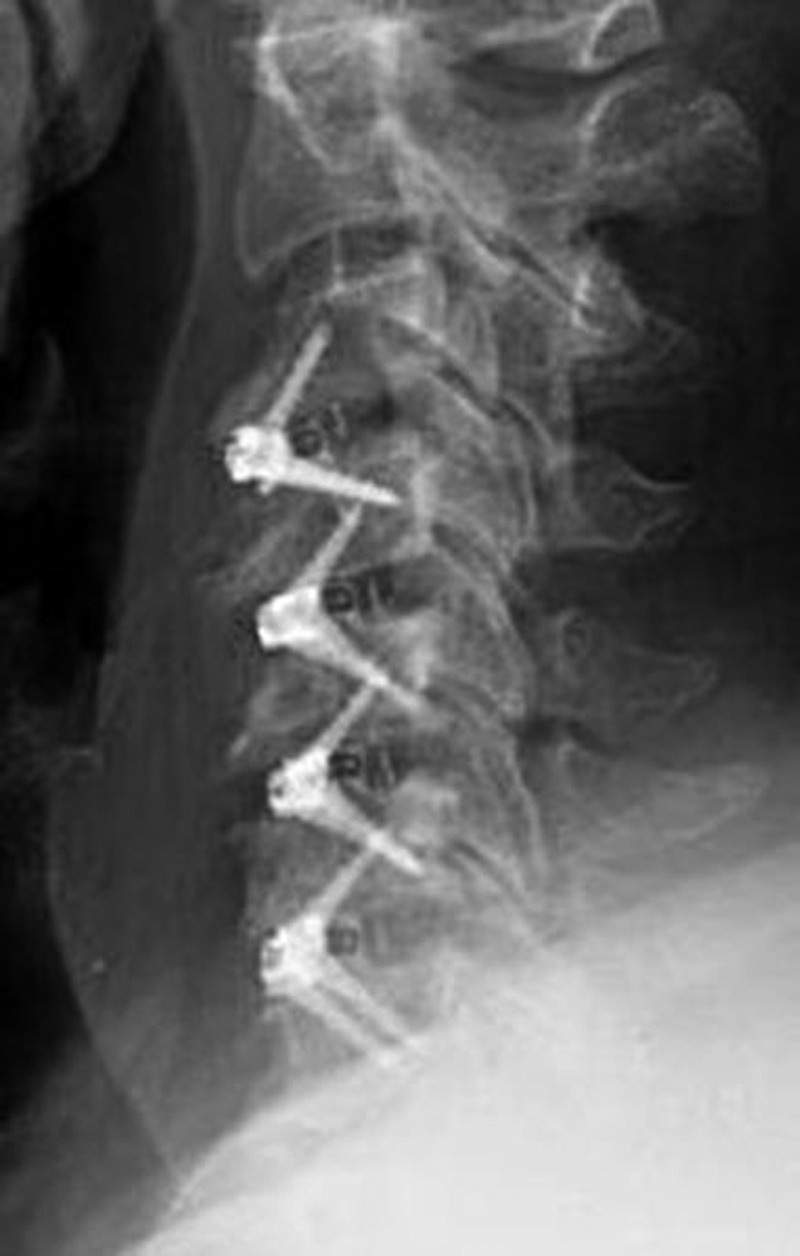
Case Example of a Four-Level ACDF Using the Optio-C Implant Sagittal radiograph at three months after surgery demonstrating preservation of disc heights.
